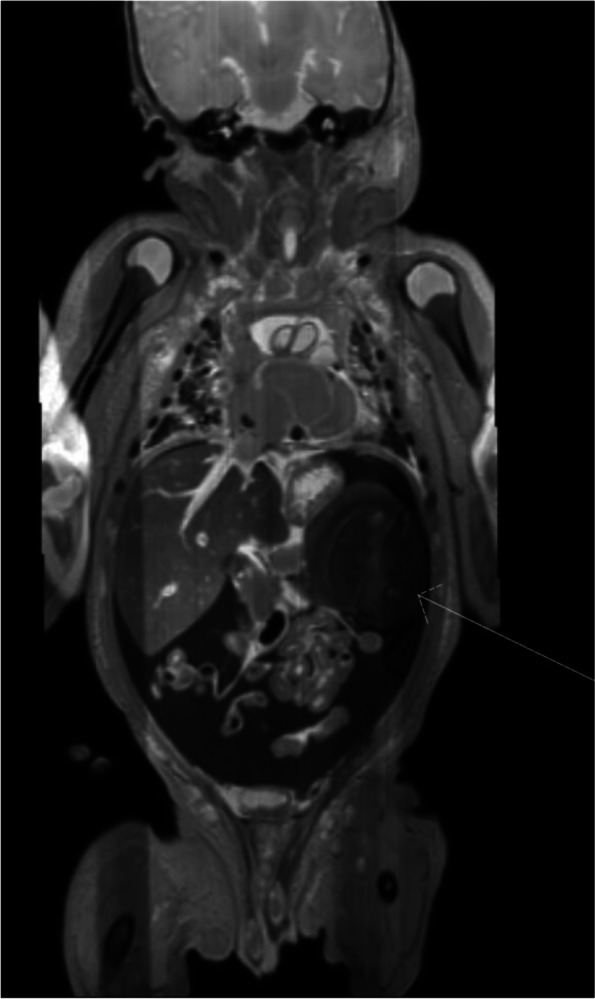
coronal T2 MR image of patient 1, performed postmortem. There is diffuse intra-abdominal blood. The arrow indicates the enlarged and inhomogeneous spleen. The image is indicative for a large splenic hemorrhage with capsular rupture
